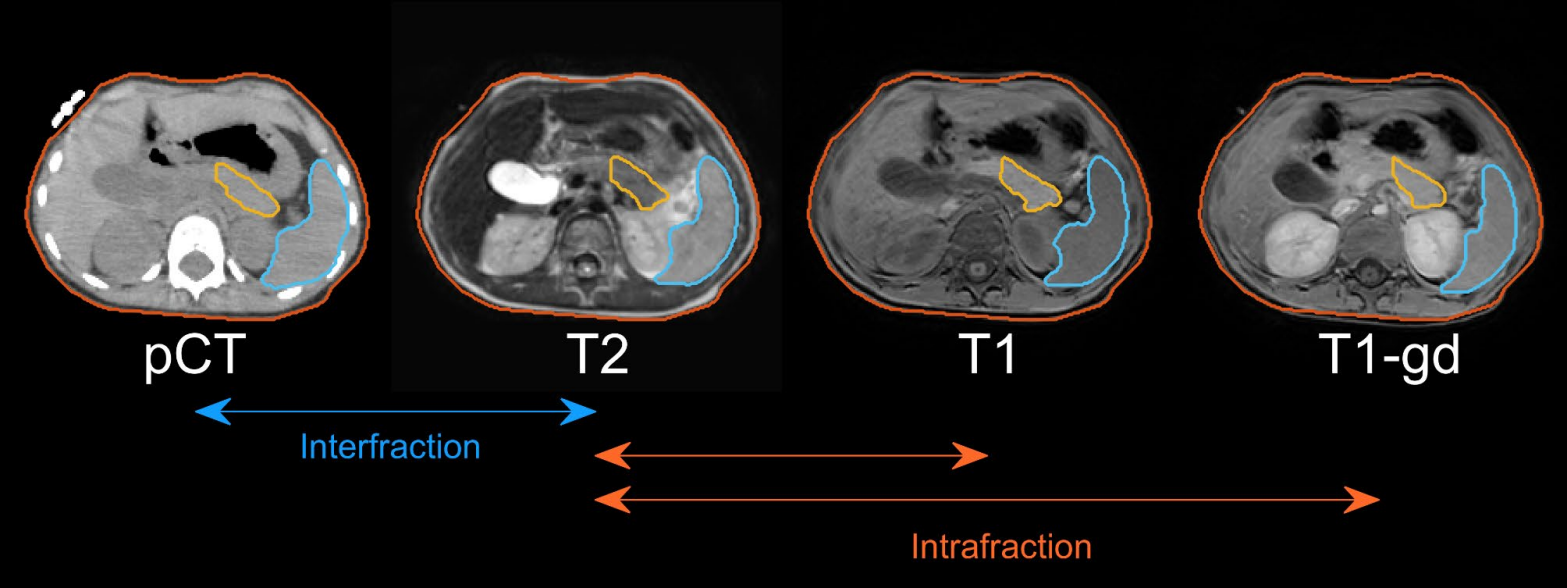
Example of registered T2, T1 and T1 with gadolinium (T1-gd) to the planning CT (pCT) with the pancreatic tail (orange) and spleen (blue) delineated. Interfraction motion is defined as positional changes between pCT and T2, whereas intrafraction motion is defined as positional changes between T2 and T1/T1-gd
